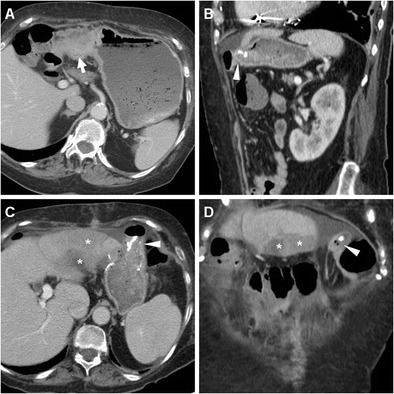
An 81-year-old male with advanced, obstructing antral cancer (thick arrow) as preoperatively depicted by CT (A) underwent palliative partial gastrectomy with Roux-en-Y reconstruction. During prolonged hospitalization the patient experience anemization and failure to thrive. Early CF (not shown) showed slow transit through EJS. On 11th POD, contrast-enhanced CT (B-D) showed some ingested materials in the remnant stomach (+), stapled EJS (arrowheads), some residual intraperitoneal air and fluid, inhomogeneous hypoattenuation of the 3rd liver segment consistent with parenchymal infection
